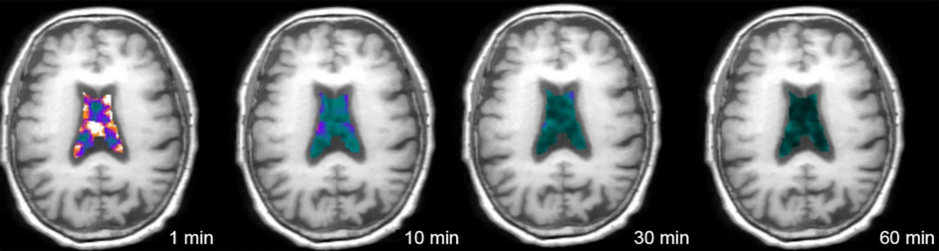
Ventricular clearance measured using dynamic PET. A single subject's PET images, superimposed over co-registered MRI, show decreasing radiotracer in lateral ventricles over the 1-h scan period. Image from Li and colleagues.^21^ MRI, magnetic resonance imaging; PET, positron emission tomography
